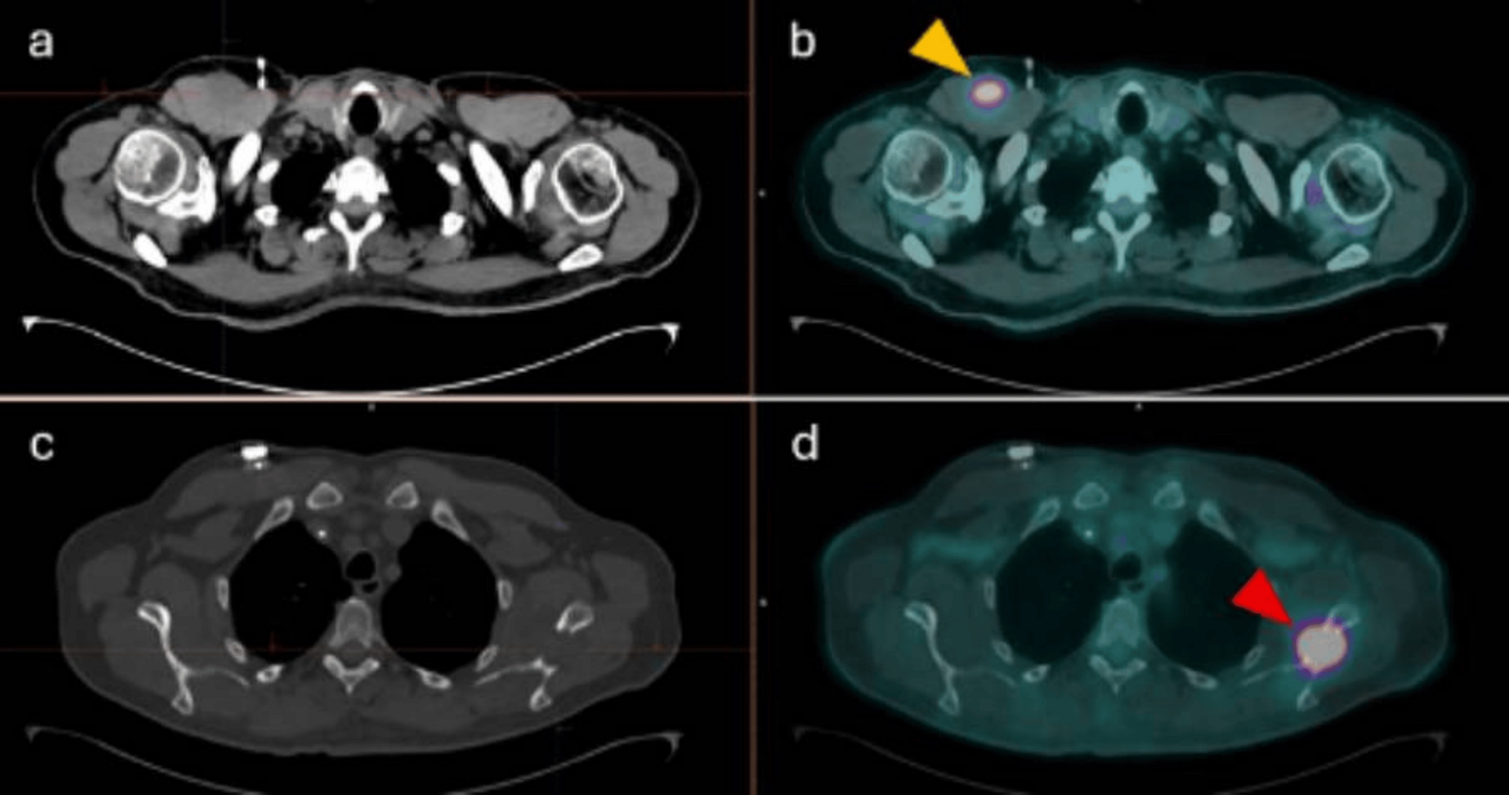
Axial CT and axial 18F-FDG PET/CT Images (a) and (b) demonstrate a hypodense, hypermetabolic lesion involving the right pectoralis major muscle (yellow arrowhead), consistent with skeletal muscle metastasis. Images (c) and (d) demonstrate a hypermetabolic, expansile, osteolytic lesion with an associated soft-tissue component involving the neck of the left scapula (red arrowhead), consistent with osseous metastatic disease. ^18^F-FDG PET/CT: ^18^F-fluorodeoxyglucose positron emission tomography with computed tomography
